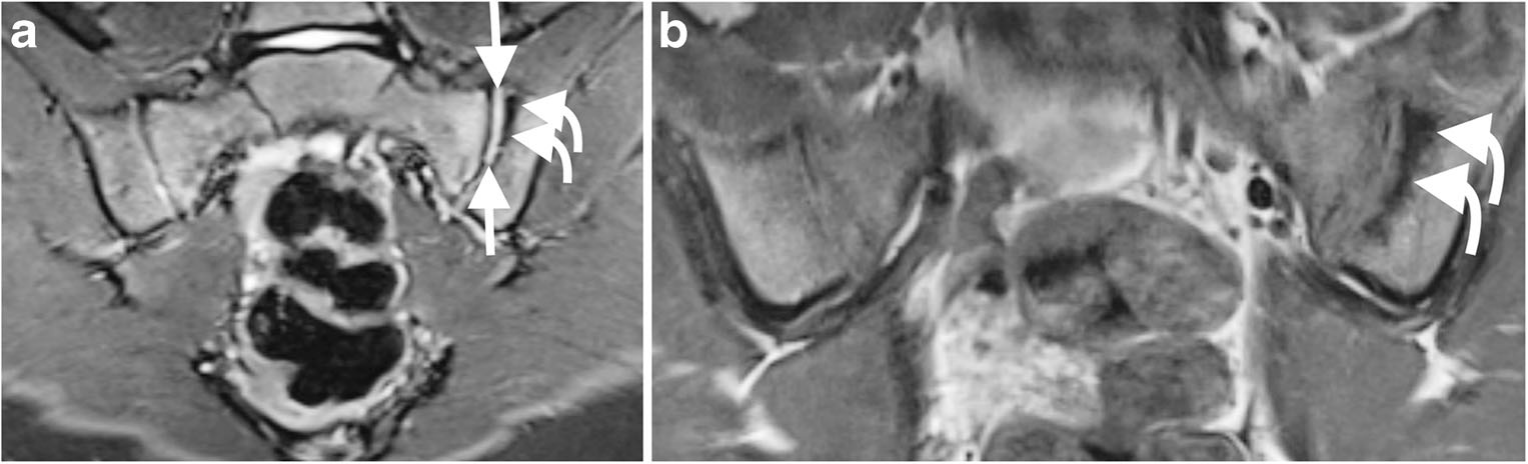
A 9-year-old girl with juvenile idiopathic arthritis and suspected sacroiliitis. a Coronal oblique short tau inversion recovery (STIR) spin echo (TR 4,000 ms, TE 36 ms) image demonstrates a left sacroiliac joint effusion, seen as high signal within the joint (*straight arrows*) and subchondral sclerosis (*curved arrows*). b T1-weighted spin echo (TR 679 ms, TE 12 ms) images also demonstrate sclerosis adjacent to the left sacroiliac joint, particularly on the iliac side of the joint (*curved arrows*)
